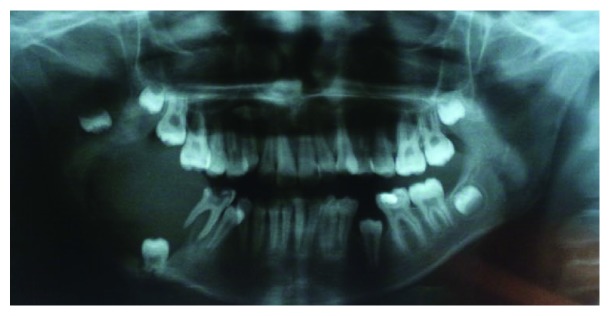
Panoramic radiographic examination revealed a unilocular radiolucent lesion extending from the body of the mandible through to the angle and right ascending ramus.
